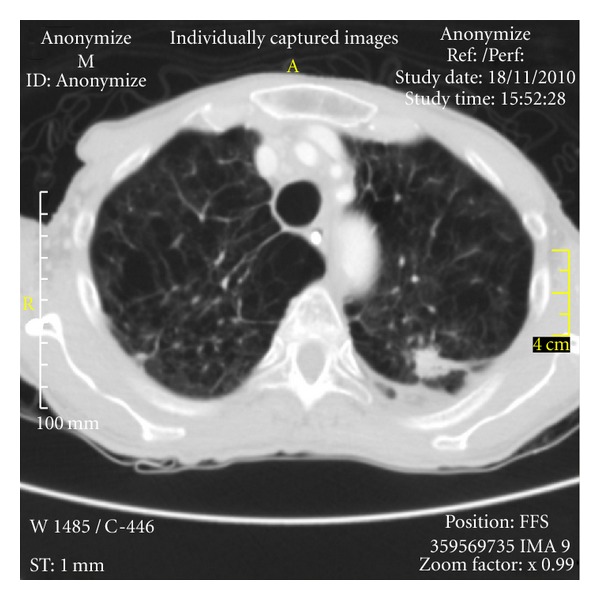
Thoracic computed tomography scan. It showed an elongated noncalcified image, with irregular contours, measuring approximately 2.8 cm × 1.4 cm, in the upper left lobe, contiguous to an area of pleural thickening, and large areas of emphysema.
